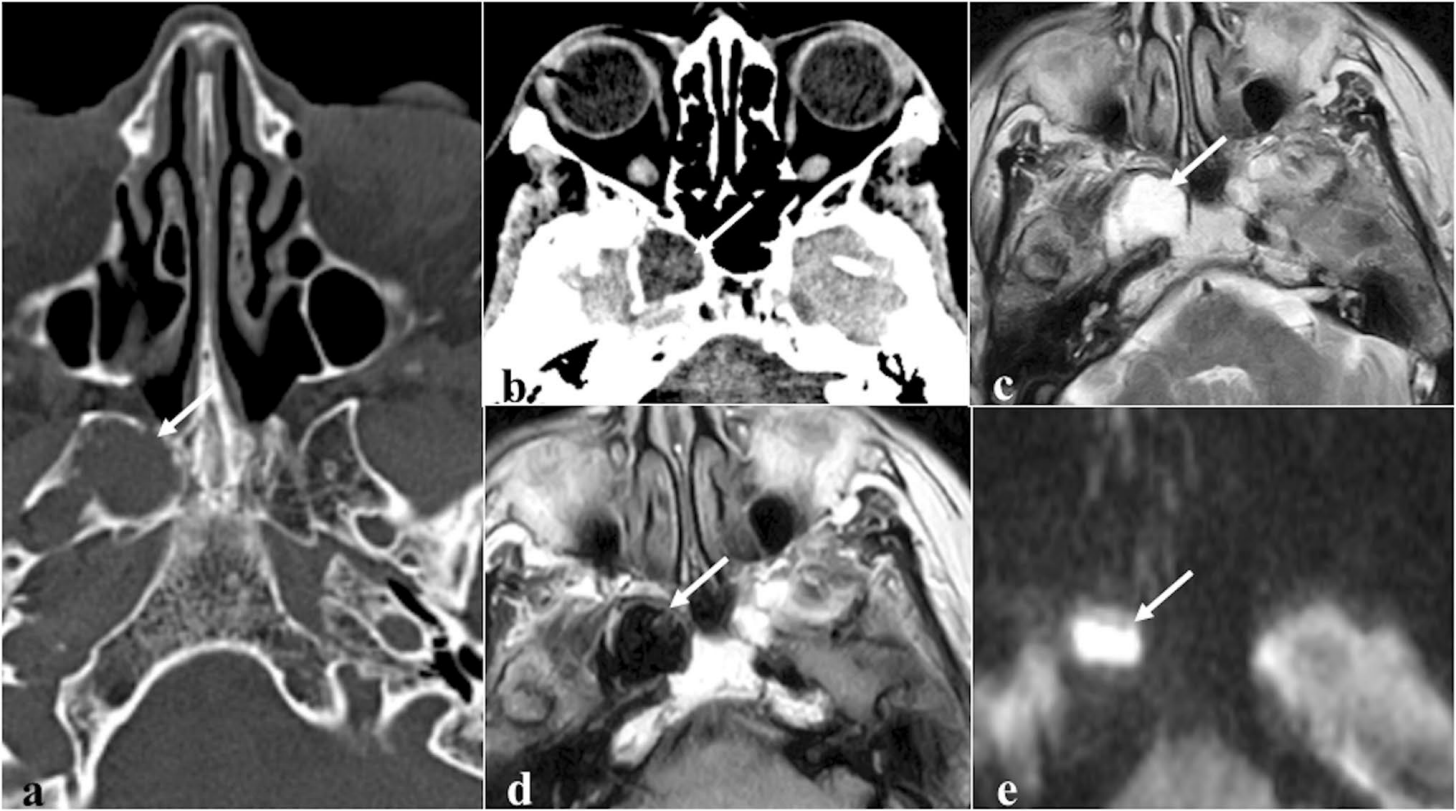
Sphenoid epidermoid cyst as an incidental finding in a 74-year-old female patient. Axial CT images (a and b) reveal a rounded lytic lesion in the right greater sphenoid wing (white arrows) with sclerotic margins and homogenous density similar to cerebrospinal fluid. Axial MRI images show that the lesion has high SI on T2W (c), heterogeneously low/dirty SI on fluid attenuated inversion recovery (d), and high SI on DWI b1000 sequences (e) due to the restricted water movements. Epidermoid cyst has similar features as arachnoid cyst on CT. Arachnoid cysts would have demonstrated homogeneous low SI on fluid attenuated inversion recovery MRI — as low as cerebrospinal fluid — and facilitated diffusion on DWI
